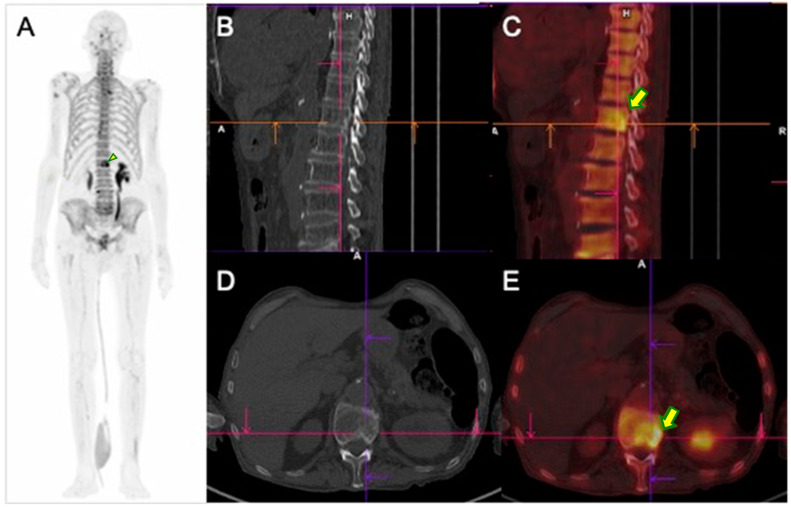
Example of PET/CT using the radiopharmaceutical ^18^F-fluoride of an 85-year-old prostate cancer patient. The patient underwent the study for restaging purposes (Gleason, 4 +3; post-prostatectomy and radiotherapy) due to biochemical recurrence (PSA = 323 ng/dL). (A). Whole-body MIP (Maximum Intensity Projection) image demonstrates a focal area of abnormal uptake in the 1st lumbar vertebrae (arrowhead). The sagittal in the CT and fused images in the sagittal (B,C) and axial (D,E) images demonstrate increased activity in areas consistent with osteoblastic bone metastasis (yellow arrows).
